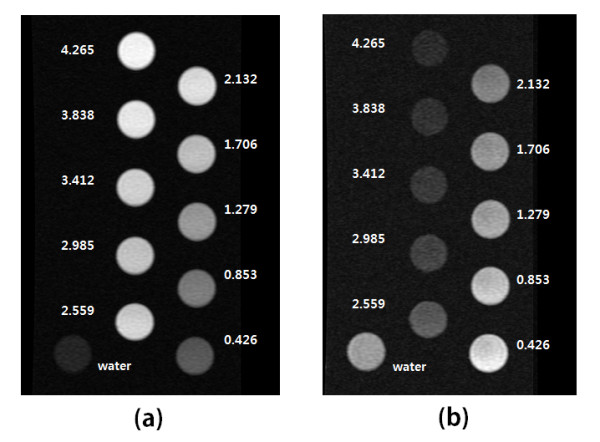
MR images for T_1 _(a) and T_2 _(b) measurements, respectively. The circular images in the picture are MR images of aqueous samples of varying concentrations with the units of mM. While a dose-dependent increase in signal intensity is seen in the *T*_1 _measurements, a dose-dependent decrease in signal intensity is observed in the *T*_2 _measurements.
